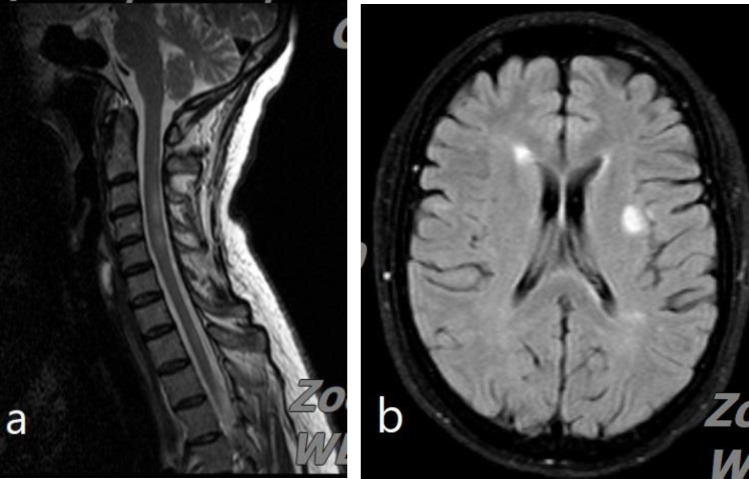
The sagittal view of the cervical and axial view of the brain MRI showed hyperintense lesions. The rest of the imagines are not shown here
